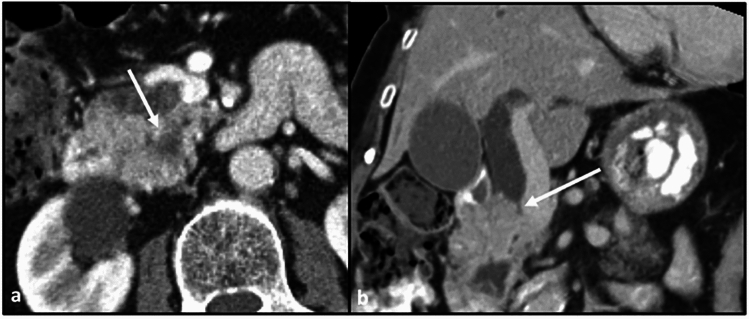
Axial CT sections with portal venous phase contrast. a Ill-defined hypodense mass in the anterior pancreatic head (arrow). b Obstruction of the common bile duct above the level of the ampulla (arrow)
